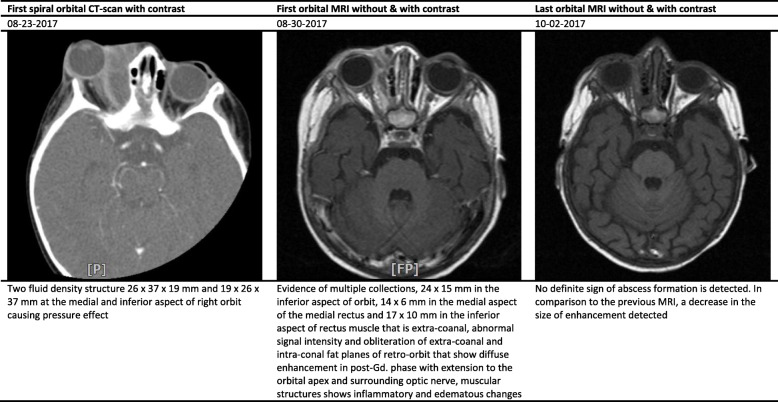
Summary findings of orbital CT-scan and MRI images at admission and during treatment in case 2
